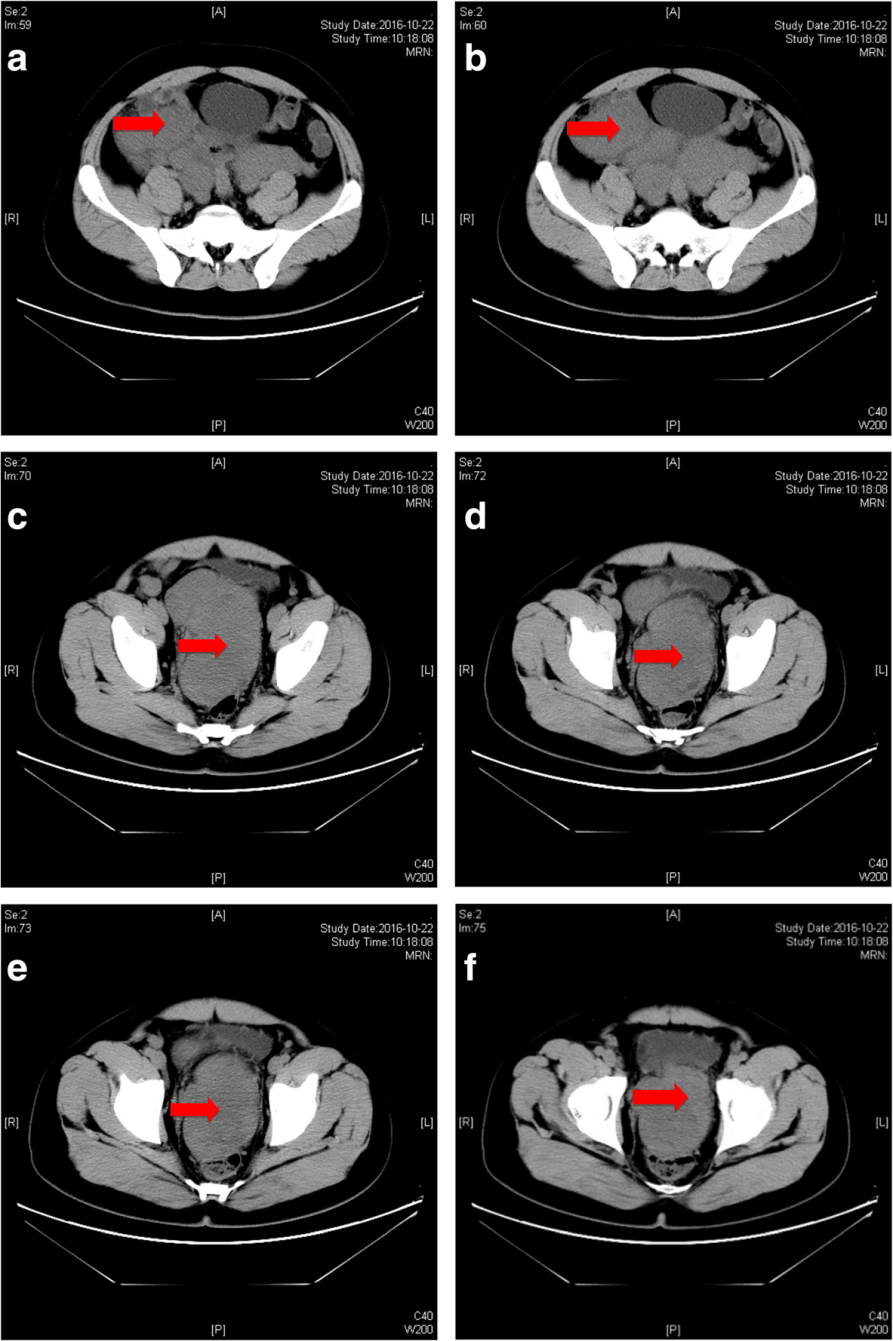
a-f Abdominal CT revealed a 13.9 × 10.6 × 17.4 cm mass between bladder and rectum
